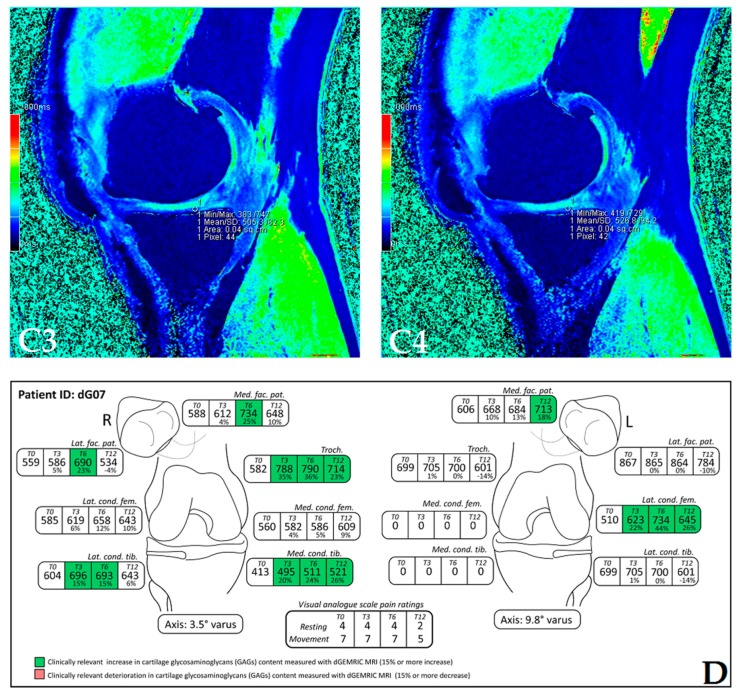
(Patient dG07) Coronal (A) and sagittal (B) MRI show complete loss of articular cartilage of the medial femoral and tibial condyles (ICRS grade IV chondromalacia), thinning and shallow fissures of the articular cartilage in the lateral femoral and tibial condyles (ICRS grade IV chondromalacia), edge osteophytes and joint effusion. The MRI with the dGEMRIC index values at four-time points (T0: baseline; T3: three months after autologous and microfragmented adipose tissue injection; T6: six months after autologous and microfragmented adipose tissue injection; T12: 12 months after autologous and microfragmented adipose tissue injection) (C1–C4). The scheme of the dGEMRIC index with different joint facets throughout the study period at T0, T3, T6 and T12 combined with VAS scale ratings in T0, T3, T6, T12 (D).
